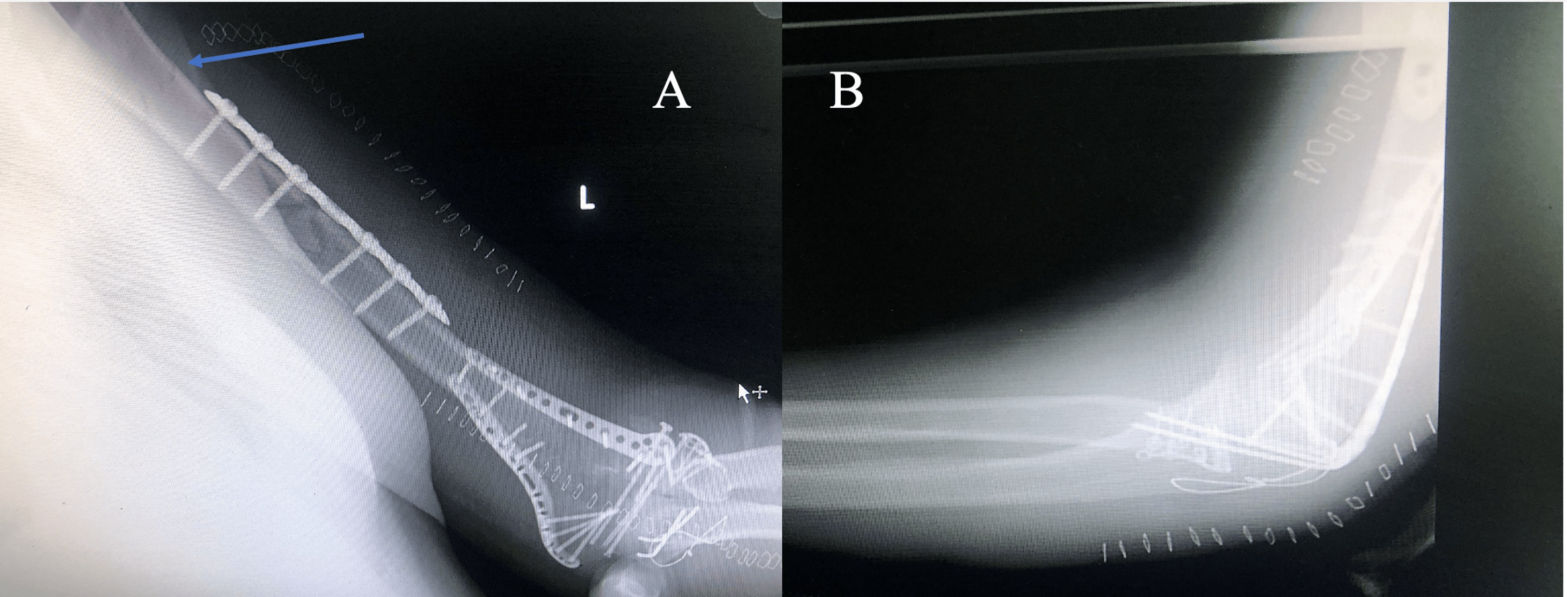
Immediate post-op anteroposterior (A) and lateral (B) radiographs of the left humerus and elbow. The untreated proximal humerus fracture is shown with the blue arrow.
